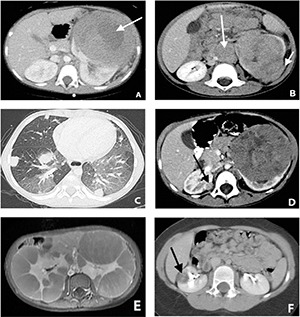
A, Large left renal mass in a 4-year old female with central areas of necrosis (arrow). Diagnosis was Wilms tumor on surgical pathology; B, Three-year old female with a left renal mass (Wilms tumor) with associated retroperitoneal lymphadenopathy (long arrow). There is also extracapsular extension of the tumor (short arrow). C, CT of the chest showed multiple metastatic pulmonary nodules; D, Five-year old female with bilateral Wilms tumor. Large left renal mass and a smaller right renal mass (arrow). Bilateral partial nephrectomies were performed; E, Nephroblastomatosis. Eighteen-month old female with an enlarged abdomen. Axial post contrast MRI shows bilateral nephromegaly containing multiple lobulated parenchymal masses with streaky enhancement; F, Six-year old female with a right renal mass (arrow). This was a renal cell carcinoma on surgical pathology after nephrectomy.
